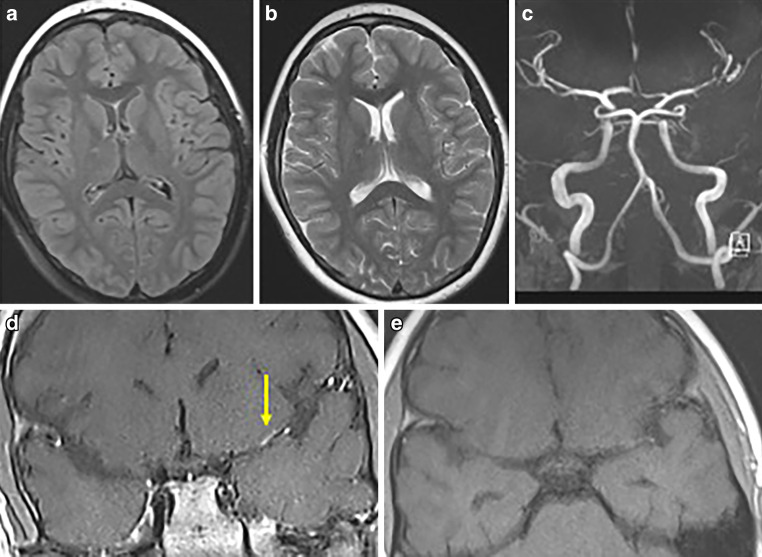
Three-month follow-up MRI after clinical onset and subsequent treatment of cerebral vasculitis due to LNB. FLAIR image (axial view; a) and T2-weighted image (axial view; b) showed no new cerebral lesions. MIP images from TOF angiography (c) demonstrated residual non-flow-limiting stenosis at the left MCA bifurcation. Fat-saturated contrast-enhanced T1-weighted images (coronal view, d; axial view, e) detected residual minimal vessel wall enhancement of the left distal M1 segment (yellow arrow, d) and no leptomeningeal enhancement
